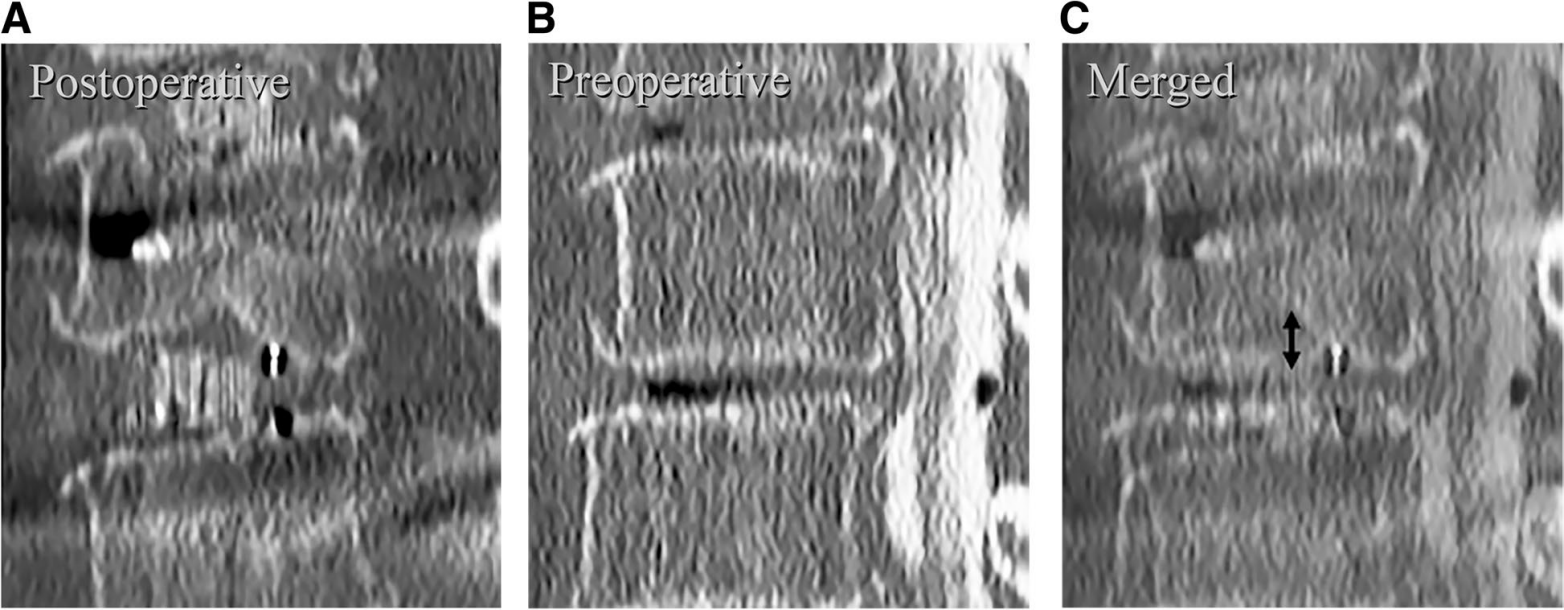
Displacement of endplate. Images of the same section in sagittal and coronal sections of multiplanar CT at 2 different time points, which are postoperative (A) and preoperative (B) were merged (C), and the minimum distance between endplate was calculated. Displacement ≥2 mm was defined as obvious
